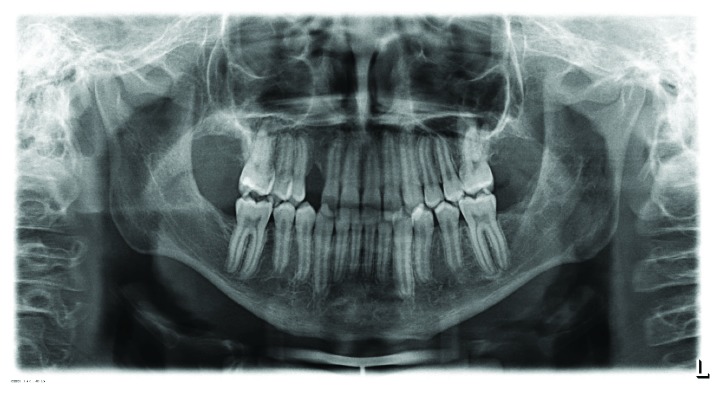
Panoramic radiograph (October 2016) 12 months after the third surgery.
